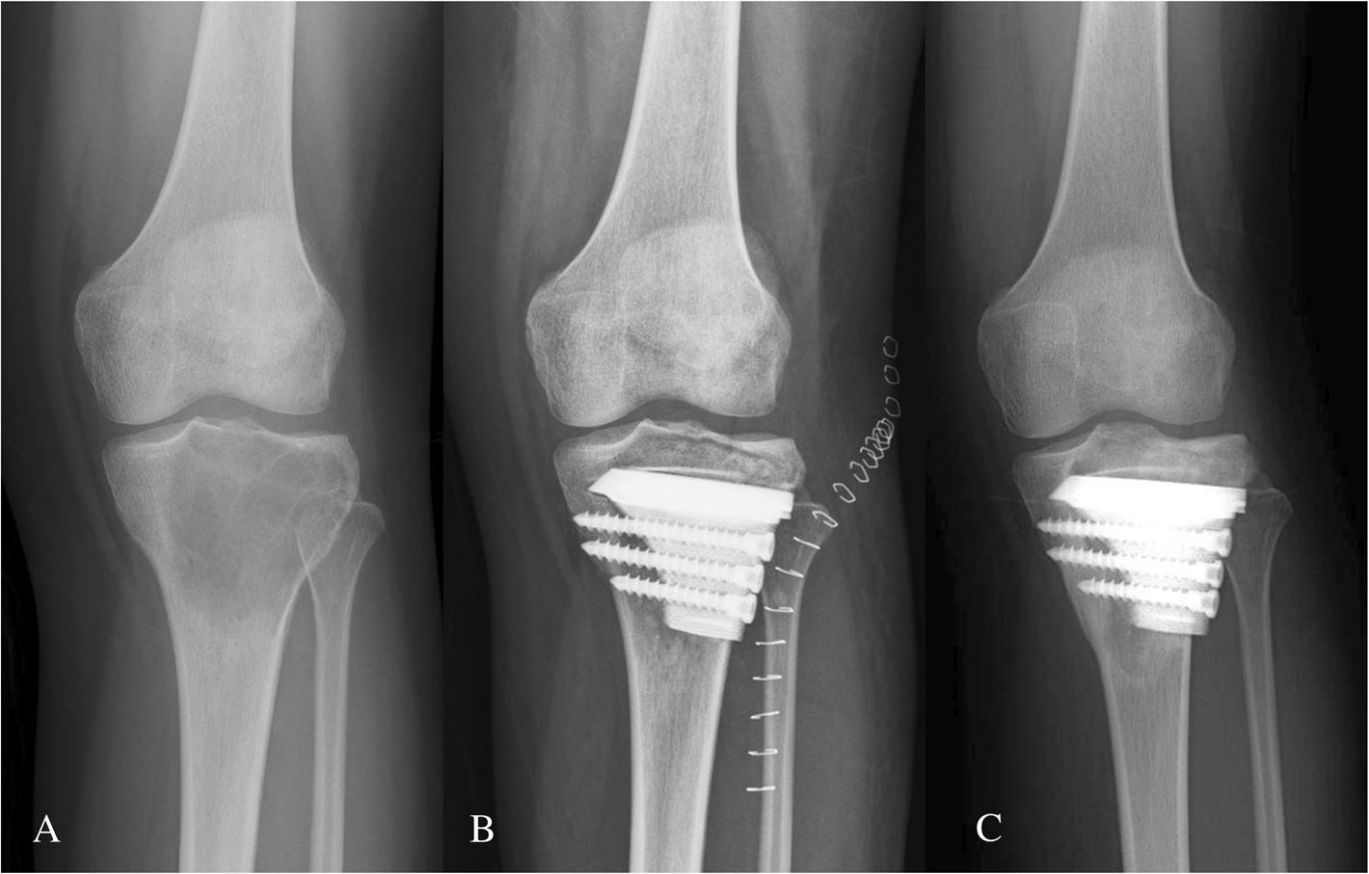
Comparison between preoperative and postoperative X-rays. a Before surgery. b One week after surgery. c One year after surgery
